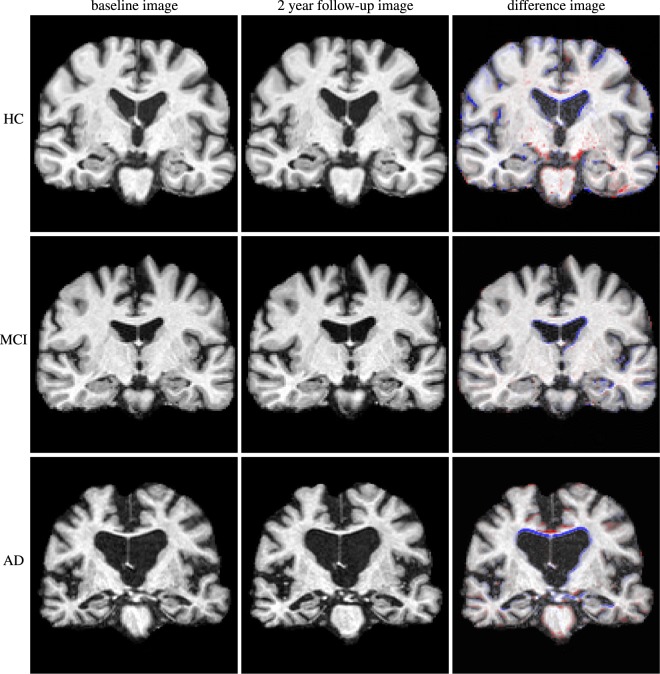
Three examples of MR images (brain-extracted) of subjects from the ADNI1 cohort in coronal section. Top row: a healthy control subject (male, 84.8 years at baseline); middle row: MCI subject (female, 71.8 year at baseline) who converted to AD after three years; bottom row: an AD patient (male, 77.5 years at baseline). Left: baseline; middle: 2-year follow-up; right: baseline with overlaid difference image of rigidly aligned images (blue: volume loss/atrophy, red: positive volume change). The differences are visually subtle, but the increased atrophy in the medial temporal lobe and the enlarged ventricles are apparent in the difference image.
